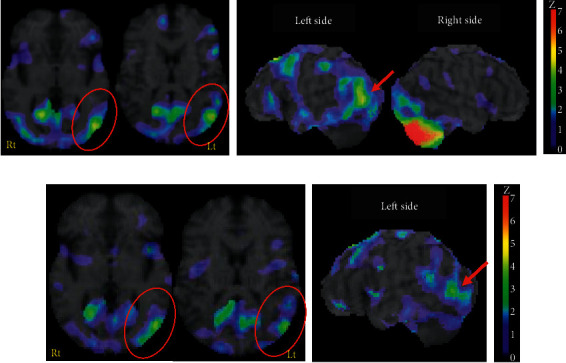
(a) A single photon emission computed tomography using ^123^I-N-isopropyl-p-iodoamphetamine (^123^I-IMP SPECT) perfusion scans on day 98. Compared with a standard normal database in perfusion findings, the *Z*-scores (SD) were calculated for each region and a regional *Z*-score of >2.0 was considered significant and indicated in green to red (compatible with the side color bar). The decreased perfusion was mainly observed in the left posterior middle frontal gyrus (pointed by red circles and arrows). (b) A following SPECT 3 years after stroke onset. The previous hypoperfusion showed an attenuation in both the area and degree on the left side view of this study.
